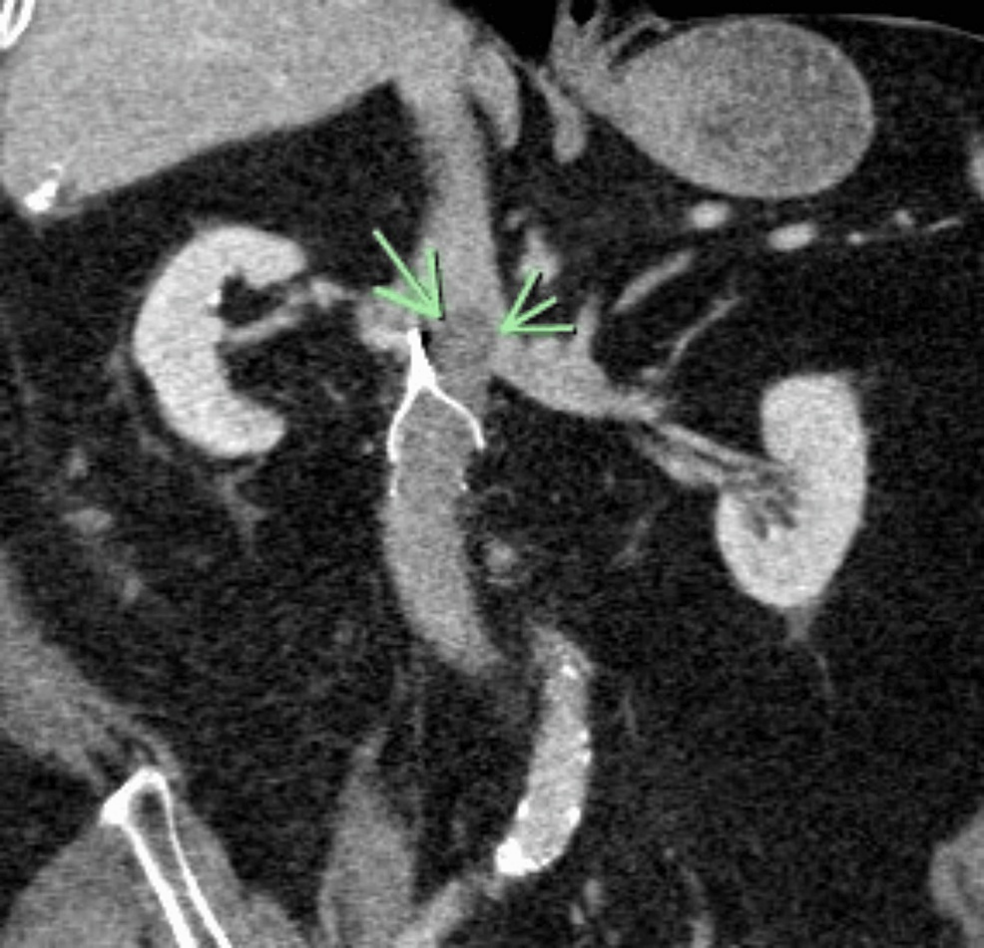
CT venography (the green arrows point to a possible thrombus positioned cephalad to the IVC filter on this coronal view). IVC: Inferior vena cava.
